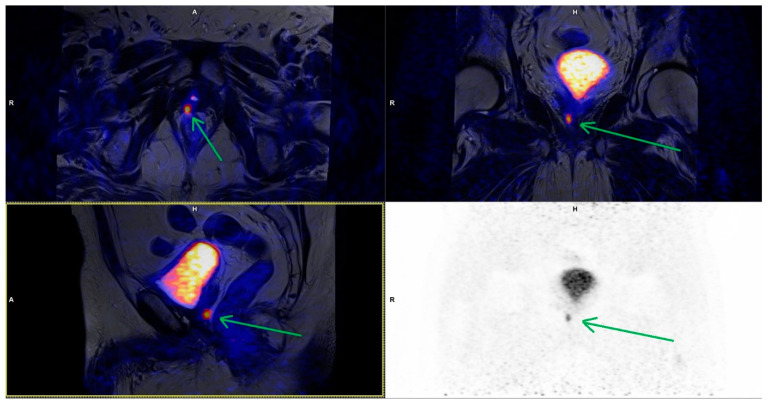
Local recurrence detected in patient with serum PSA level 0.27 ng/mL. The lesion is located to the right of the subvesical part of the urethra (green arrow). T2_tse_bl MR images fused with PET (upper images and lower left) and PET AC images (lower right).
